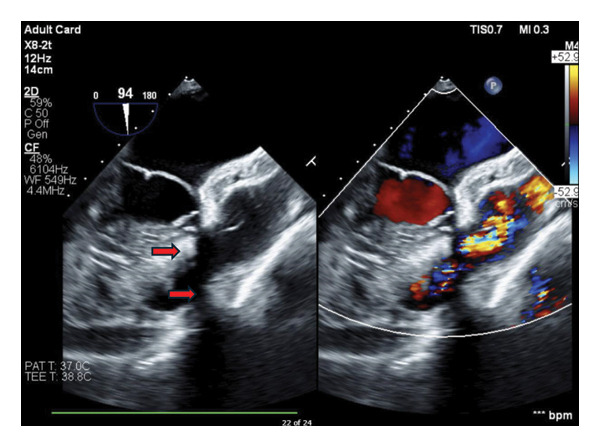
View of the RVOT on TEE demonstrating subvalvular RVOT muscle bands (marked with red arrows) and flow acceleration on color Doppler.
